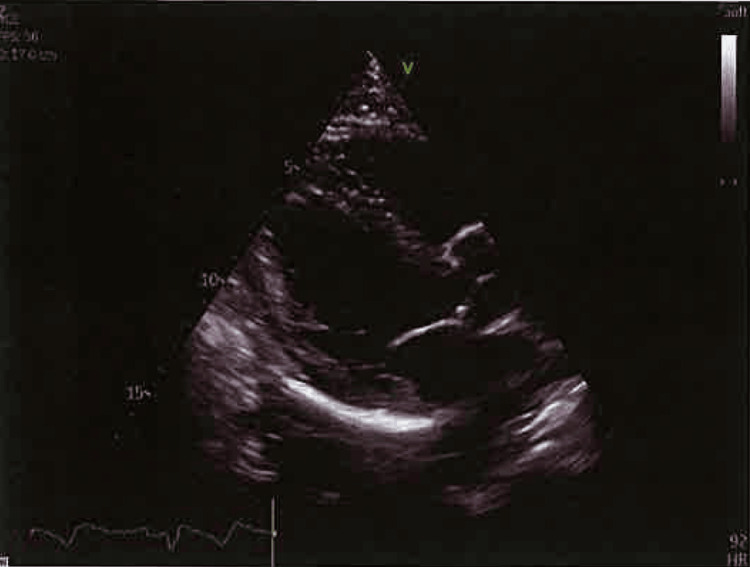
Echocardiogram, showing no signs of endocarditis
